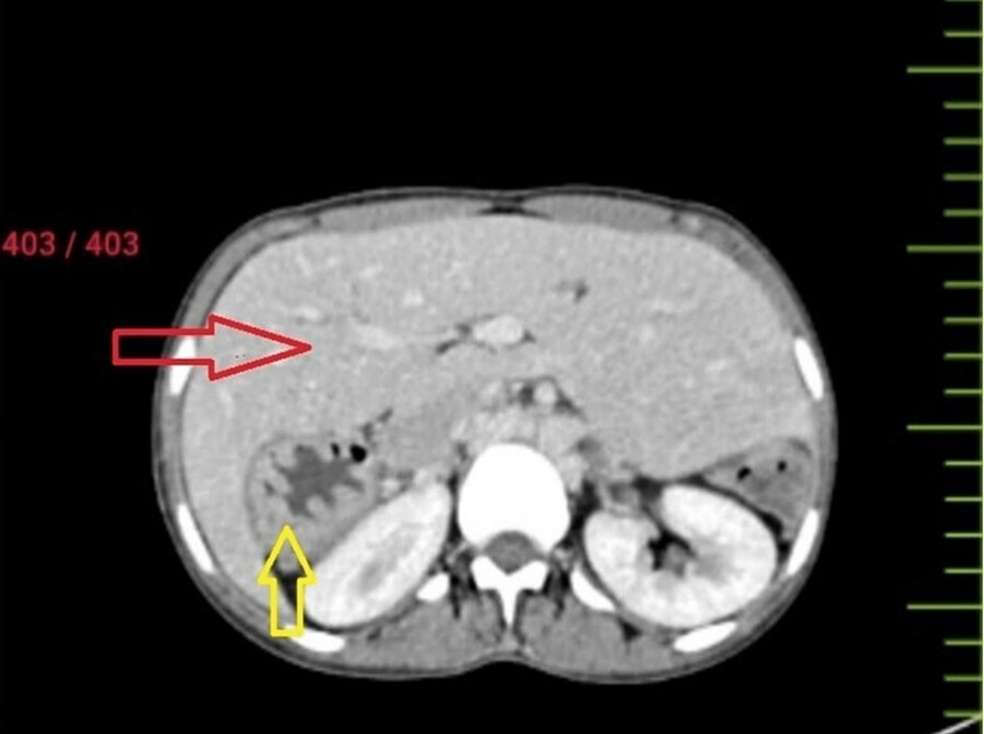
The red arrow showing the central large liver extending from the right hypogastrium to the left hypogastrium on CT angiography, and the yellow arrow showing the spleen in the right iliac fossa (wandering spleen) on CT angiography. CT, computed tomography
